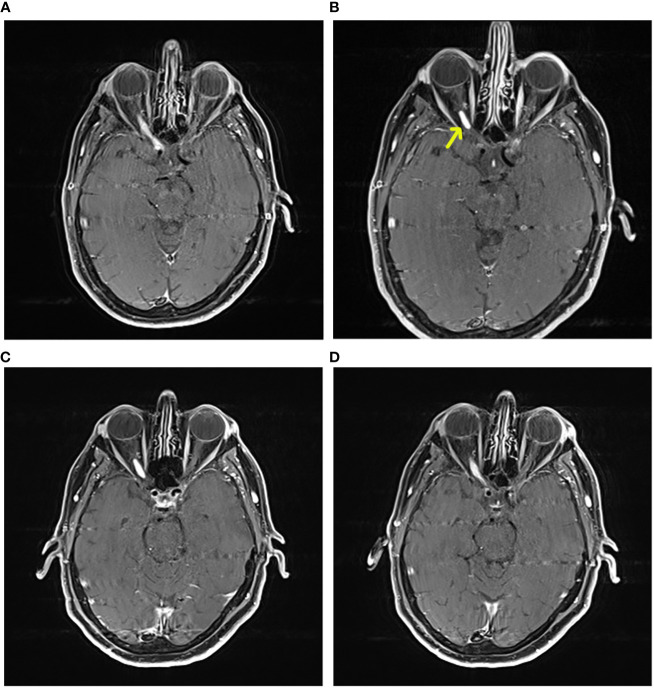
Axial contrast enhanced T1 MRI. A 56-year-old male VHL patient followed in our clinic with a right optic nerve hemangioblastoma. The patient experienced acute visual field defects in the right eye. Fundoscopy was normal. MRI showed a large right intraorbital optic nerve hemangioblastoma without edema (yellow arrow). Corticosteroids were not effective for reducing symptoms. Because surgical resection was unfeasible and was anticipated to lead to total blindness of the right eye, the patient was not operated – to cherish a couple more “good” years. Surgery will be planned as soon as peritumoral edema develops and threatens the contralateral eye. Tumor volume has now remained stable for 4 years. (A) time at diagnosis, tumor length 13mm and width 4mm. (B) 20 months later, tumor length 31mm and width 6.7mm (= growth); note progression towards optic chiasm, and less intense contrast enhancement, compared to (A, C): also 20 months, but shorter image acquisition time after contrast administration, now there is no growth compared to (A, D): longer image acquisition time after contrast administration, tumor length 24mm, width 6mm, there is growth compared to (A) Note peritumoral contrast leakage along optic nerve tract, similar to (B).
